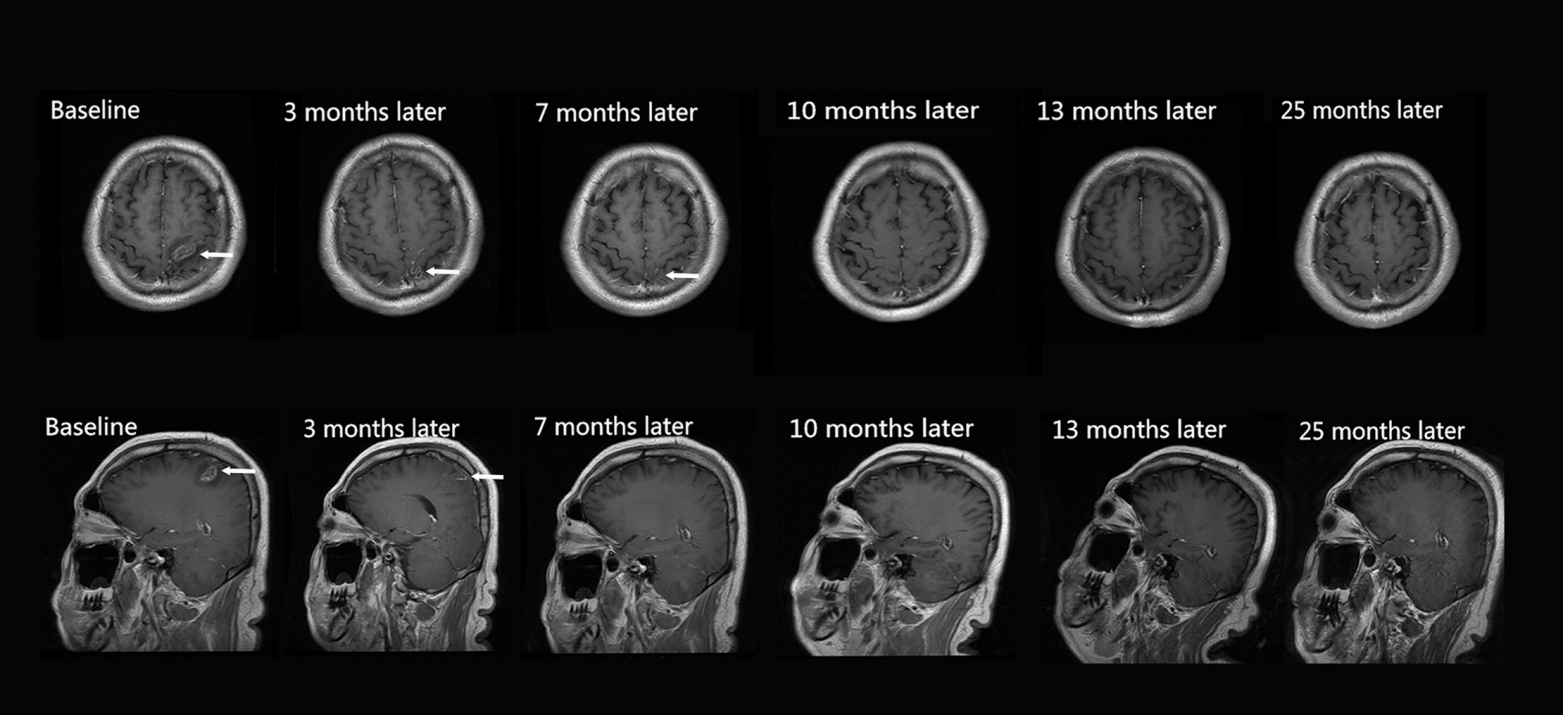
Axial and sagittal images of a 45-year-old male patient with a 3-month history of right hemiparesis. Case 6. An axial and sagittal T1 FLAIR image shows that there are serpiginous and irregular enhanced lesions in the left frontal and parietal lobes (white arrows) at baseline MRI. After 2 courses of therapy (10 months later) and follow-ups for 25 months, the left frontal and parietal lobe lesions disappeared completely, and no stable, chronic lesions occurred.
